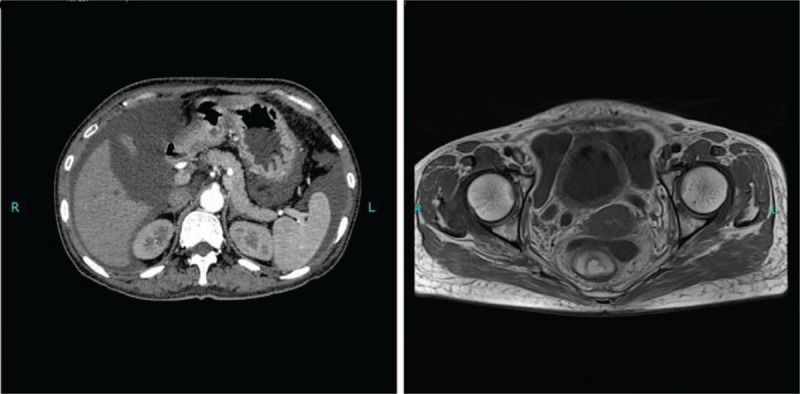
CT scan of the abdomen showing massive ascites, no tumor lesion found in CT scan of the abdomen and pelvic MRI. CT = computed tomography, MRI = magnetic resonance imaging.
